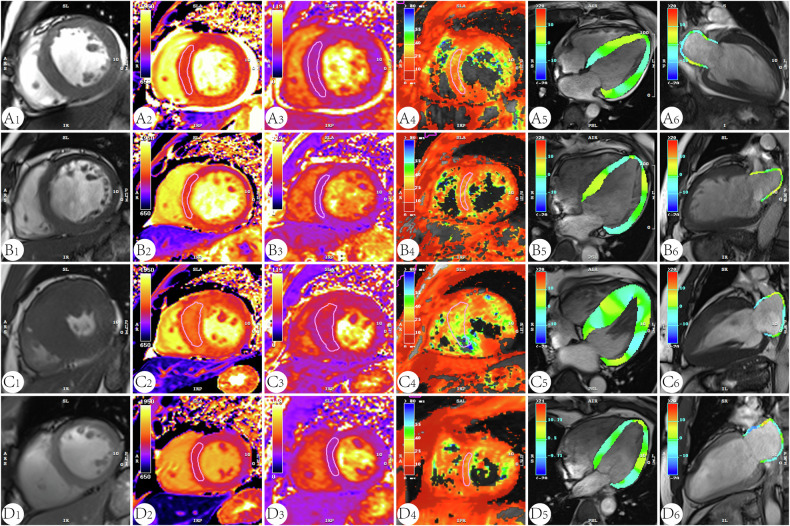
Quantitative cardiac MRI measurements in a UC patient (A), an HTN patient (B), an HCM patient (C), and a healthy subject (D). A_1_, B_1_, C_1_, and D_1_ a single short-axis stack; A_2–4_, B_2–4_, C_2–4_, and D_2–4_ quantitative CMR measurements of native T1, T2, and T2* series; A_5_, B_5_, C_5_, and D_5_ 4-chamber view of LA systolic phase; A_6_, B_6_, C_6_, and D_6_ 2-chamber view of LA systolic phase (the LA color varies with different phases and strain parameters)
